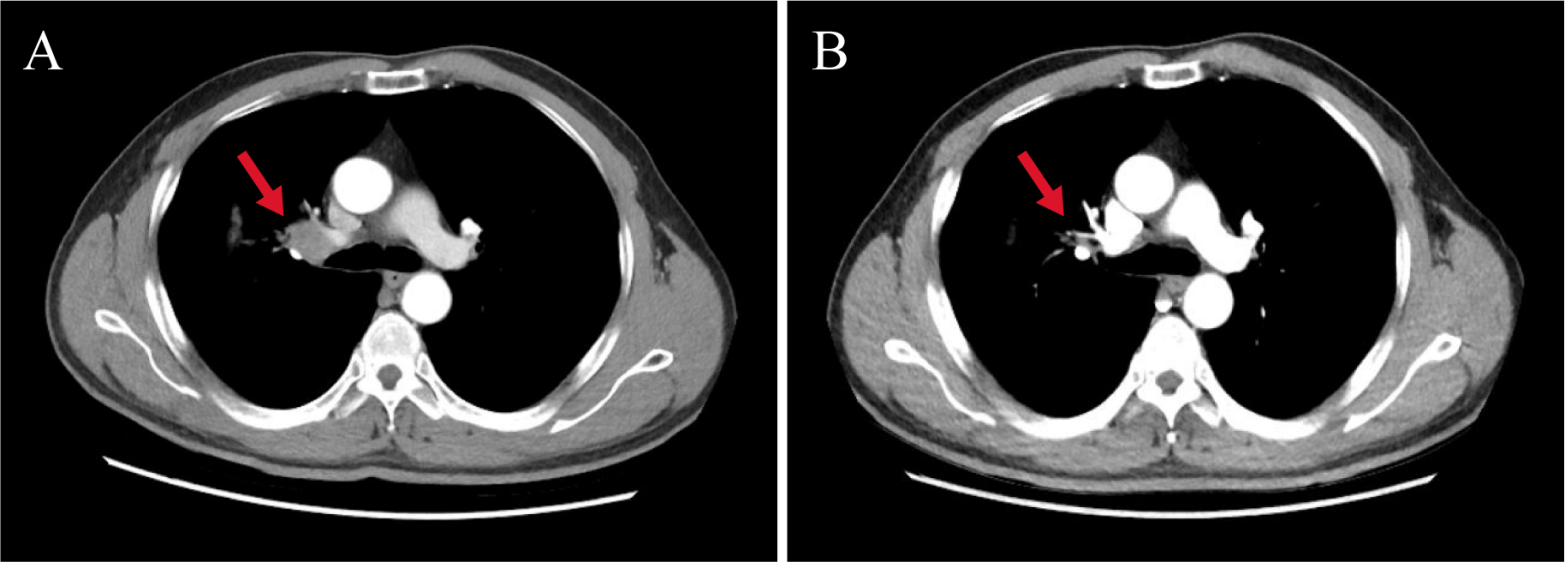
Changes in target lesion size during treatment. (A) CT scan with chest contrast (before immunotherapy). (B) CT scan with chest contrast (after 4 months of sugemalimab treatment). The red arrows in the figure mark the target lesions of enlarged lymph nodes. Chest imaging showed a reduction in the size of the hilar lymph nodes from 2.3 cm to 1.4 cm after four cycles of treatment, with an assessment of PR at the end of treatment.
